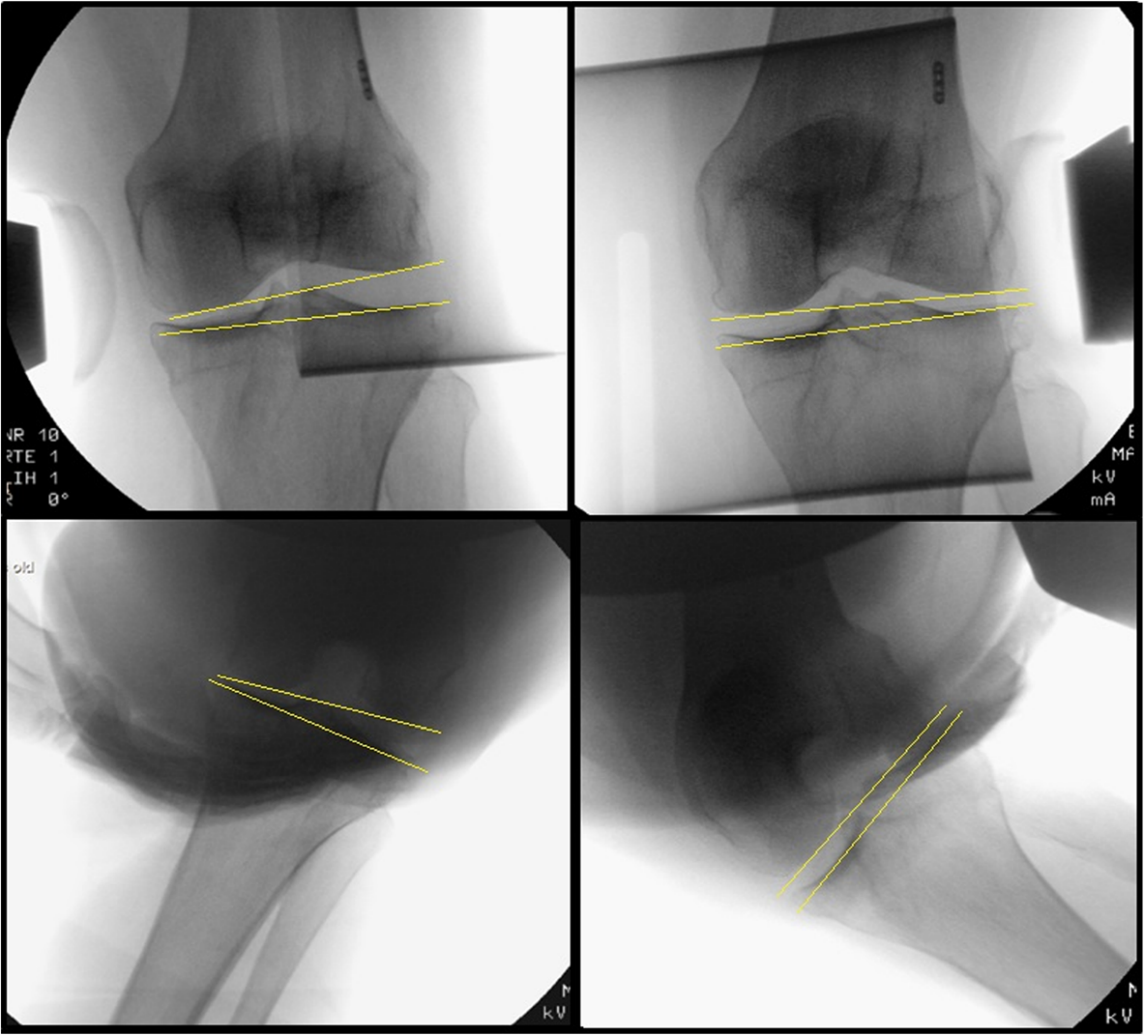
Valgus and varus laxity stress radiographs with joint space opening. Subscript: Pre-operative stress radiographs of the knee in 30° and 70° of knee flexion in an anaesthetized patient. The upper two figures show radiographs in 30° of flexion with varus (left) and valgus (right) stress. The lower two figures show radiographs in 70° of flexion in varus (left) and valgus (right) stress. The angle between a tangent line on the femur condyles and a line through the deepest tibial joint surfaces was determined and compared to the natural (unstressed) knee joint line congruence angle
